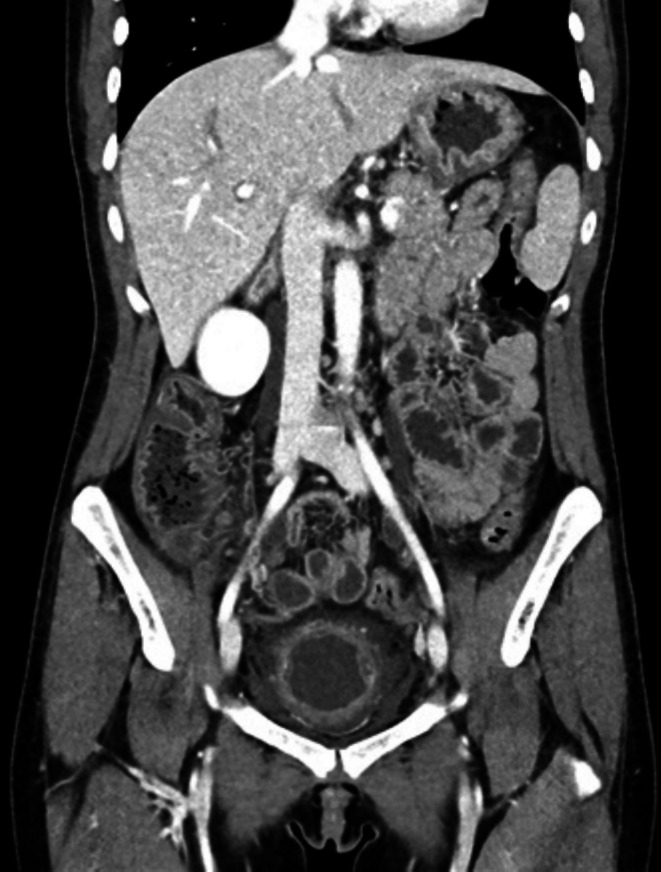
CT scan after therapy: decreased mural thickening, decreased ascites, and decreased bladder wall edema.
